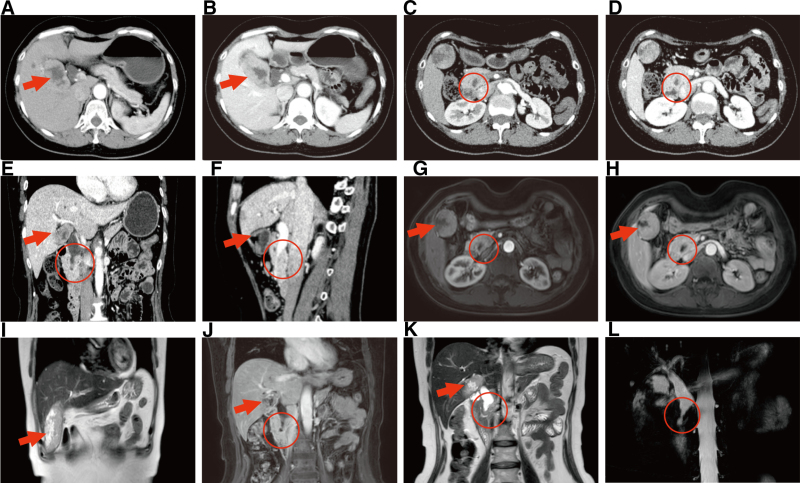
The results of the patient’s CT and MRCP. (A,B) Preoperative CT scans in the arterial (A) and venous phases (B) revealed irregular thickening of the gallbladder wall and the formation of a soft tissue mass protruding into the gallbladder cavity. The cavity was filled with irregular masses, and the boundaries were unclear. (C,D) Preoperative CT scans in the arterial (C) and venous phases (D) revealed a mass at the distal end of the common bile duct, with dilation of the proximal part. (E,F) The coronal (E) and sagittal (F) views in the CT scan indicated a space-occupying lesion within the gallbladder cavity, as well as stenosis of the distal common bile duct and dilation of the proximal common bile duct. (G,H) The MRI arterial (G) and venous phases (H) indicated an enlarged gallbladder volume. Irregular nodular thickening was observed on the base and body wall of the gallbladder, with soft tissue masses protruding into the gallbladder cavity. After enhanced scanning, the masses showed heterogeneous enhancement. (I) The MRI coronal view shows a lesion within the gallbladder. (J–L) The MRI coronal scan (J,K) and MRCP (L) indicate that the distal end of the common bile duct is narrowed, while the proximal part is dilated. The arrow indicates the lesion of the gallbladder. The circular mark indicates the lesion of the common bile duct. CT = computed tomography, MRCP = magnetic resonance cholangiopancreatography, MRI = magnetic resonance imaging.
